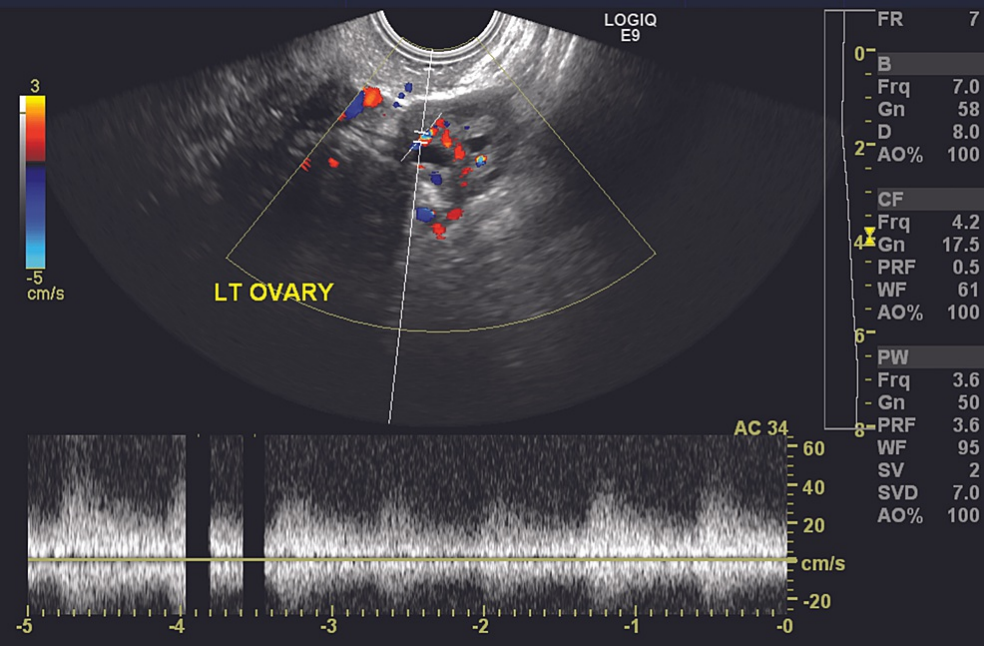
Transabdominal ultrasound revealing a normal appearing left ovary with normal blood flow on color Doppler
